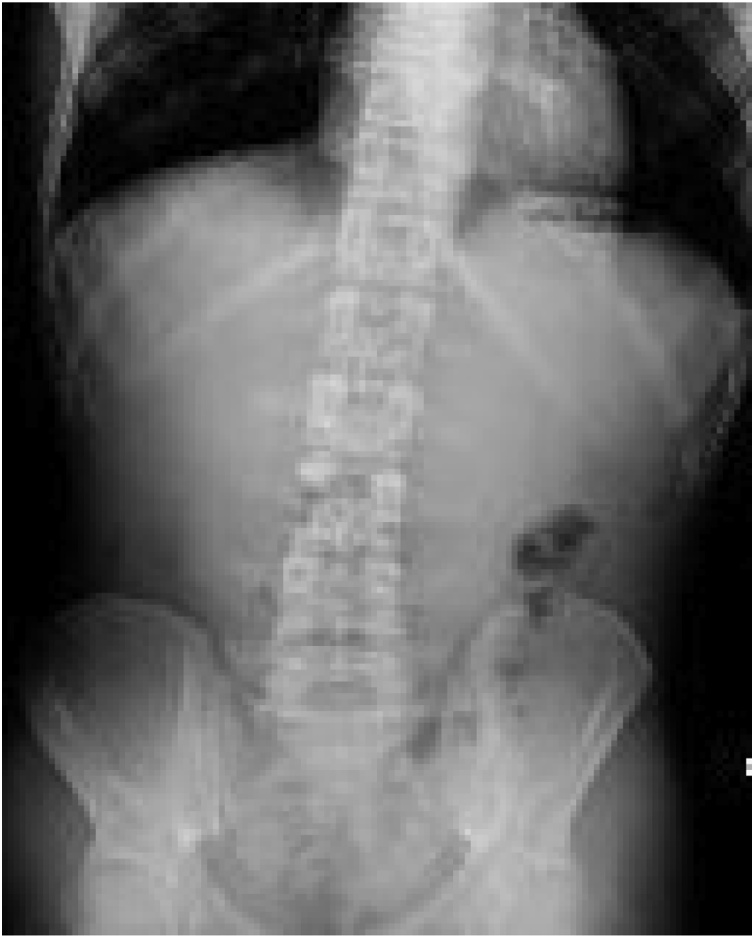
Abdominal x-ray unremarkable except for a decrease in bowel air content.
